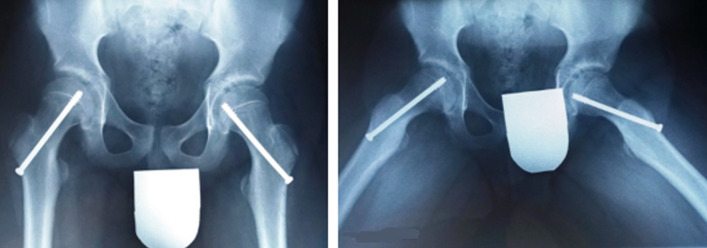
incidence radiographique de face et de profil des deux hanches à J1 post opératoire, confirmant la position du matériel d’ostéosynthèse dans les deux hanches
